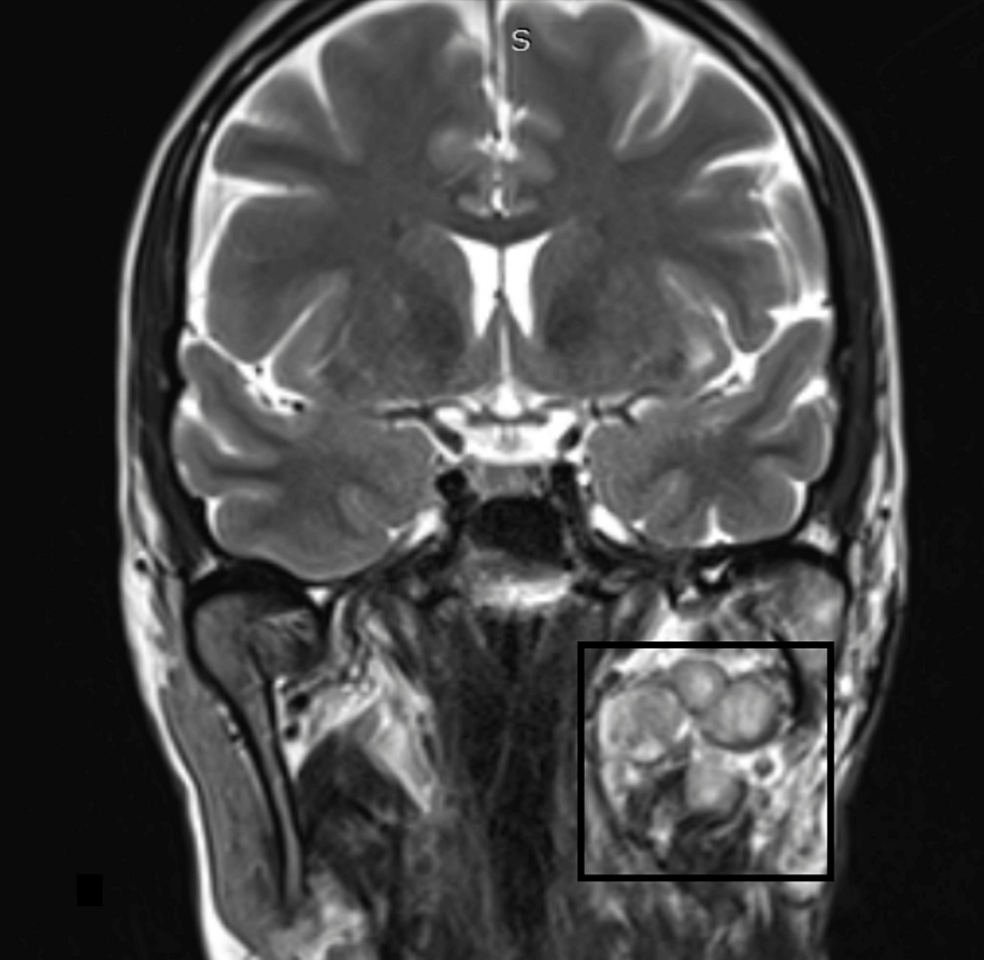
MRI coronal view revealing the multinodular recurrence of the pleomorphic adenoma (black square)
